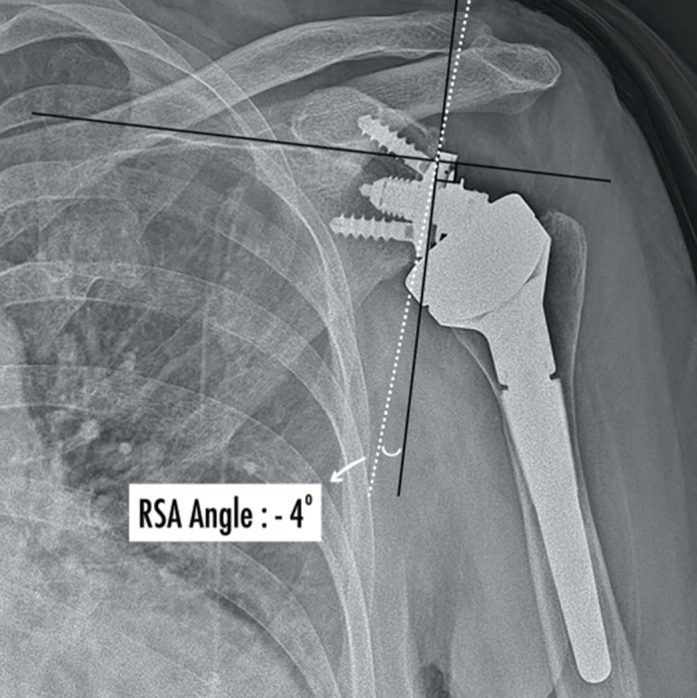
Postoperative X-ray demonstrate measurement of the RSA angle in medialized inlay prosthesis. RSA, Reverse shoulder arthroplasty.
